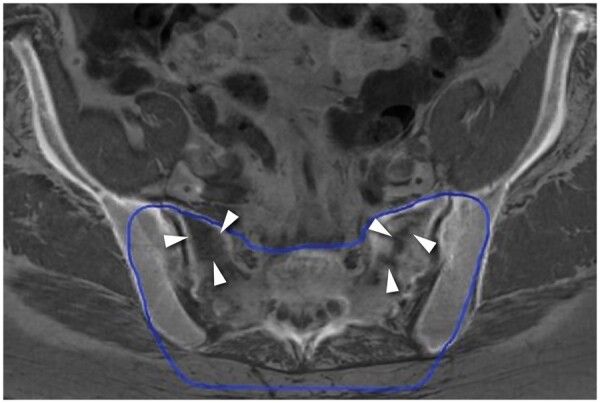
Superimposed MR scan and treatment planning CT scan of a patient with IF (white arrowheads) occurring within the low-dose volume (blue line). CT = computed tomography; IF = insufficiency fractures; MR = magnetic resonance.
